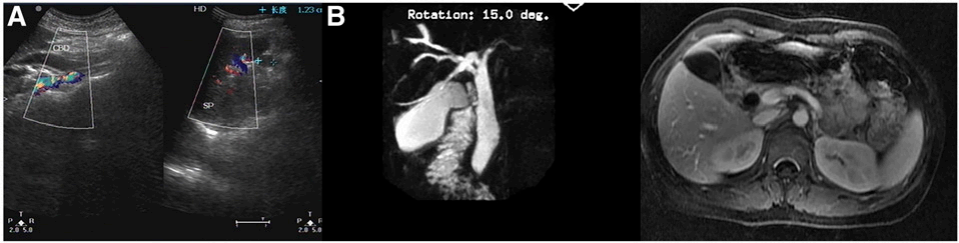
(A and B) Ultrasonography and magnetic resonance cholangiopancreatography showing dilatation of the common bile duct. This figure appears in color at www.ajtmh.org.
